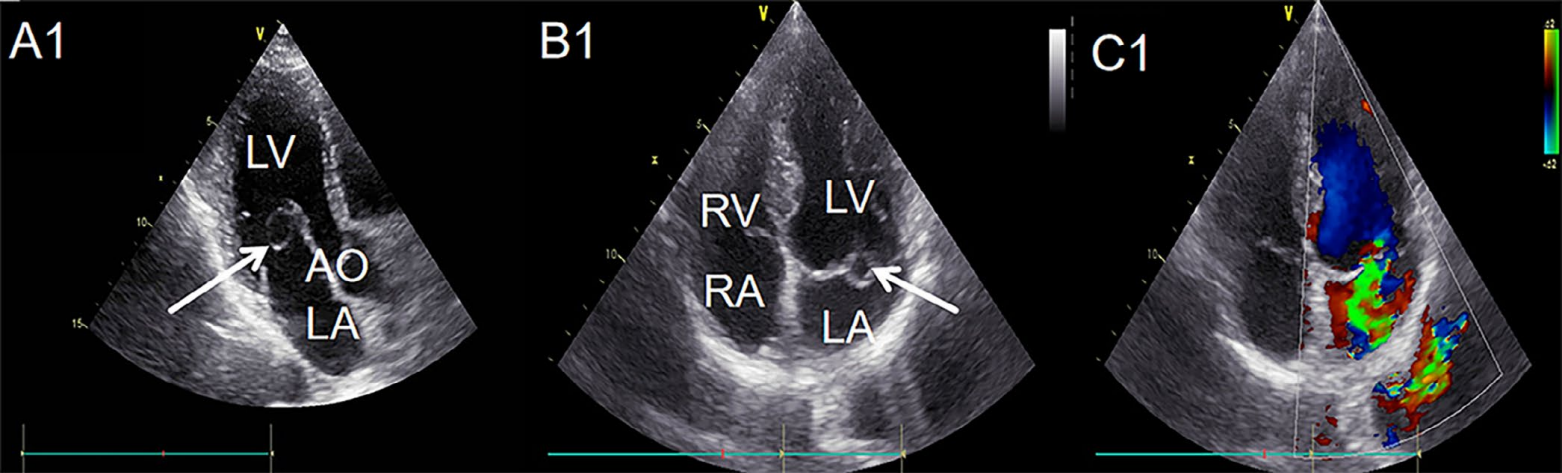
The features of case 2 on TTE and color Doppler. From the figure (A1, B1), it can be clearly seen that there is a small, round mass above the posterior part of the mitral valve, with a smooth boundary (shown by the white arrow in the figure). Color Doppler (C1) shows mitral regurgitation. LA, left atrium; LV, left ventricle; RA, right atrium; RV, right ventricle.
